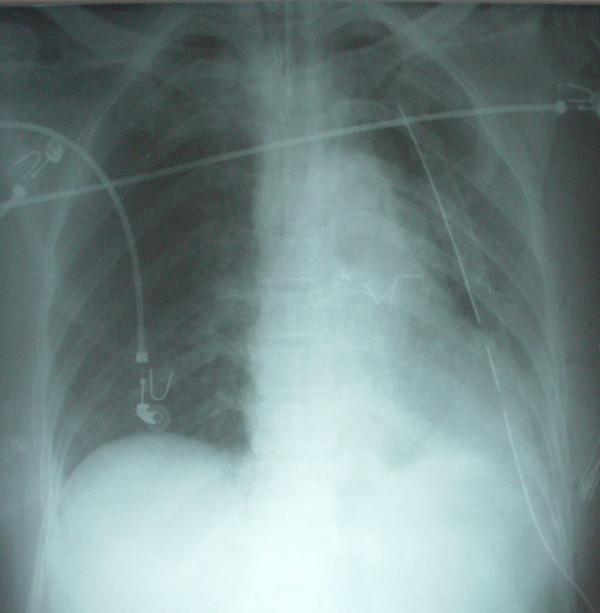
Early postoperative chest radiography showing a normal appearance.
